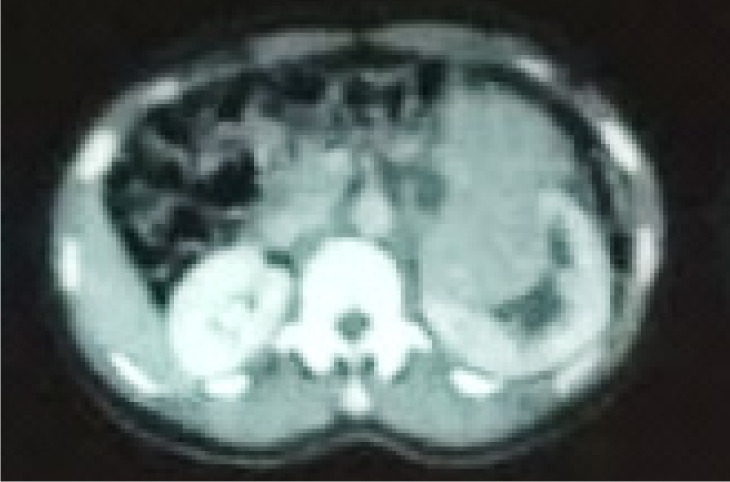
Abdominal CT scan showing a suspicious tissue mass in the left kidney.
